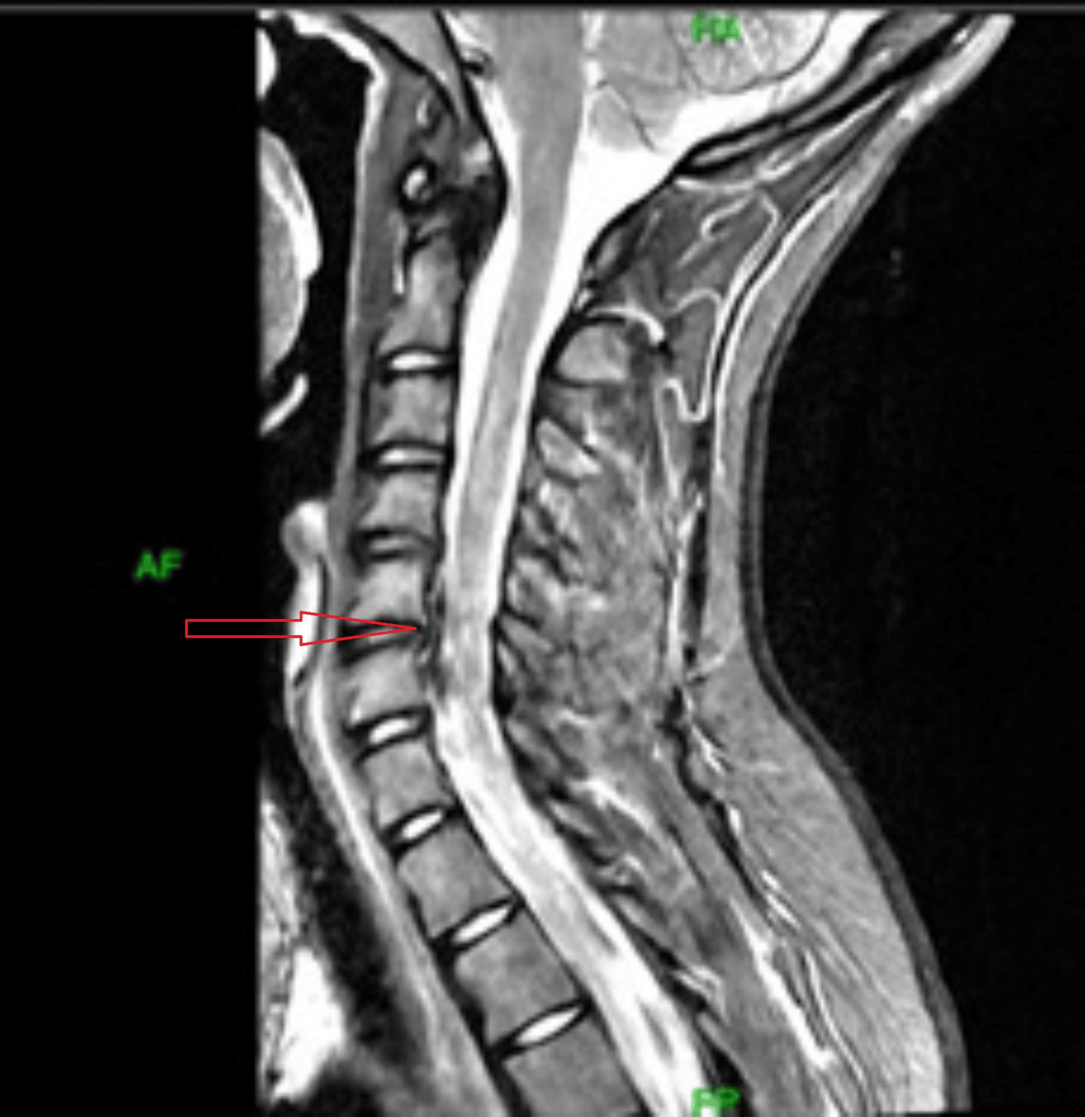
MRI of cervical spine: sagittal view. MRI of the cervical spine shows minimal degenerative changes - presence of osteophytes at the C5 and C6 posterior vertebral endplates indicated by the red arrow, without significant foraminal or spinal canal stenosis.
